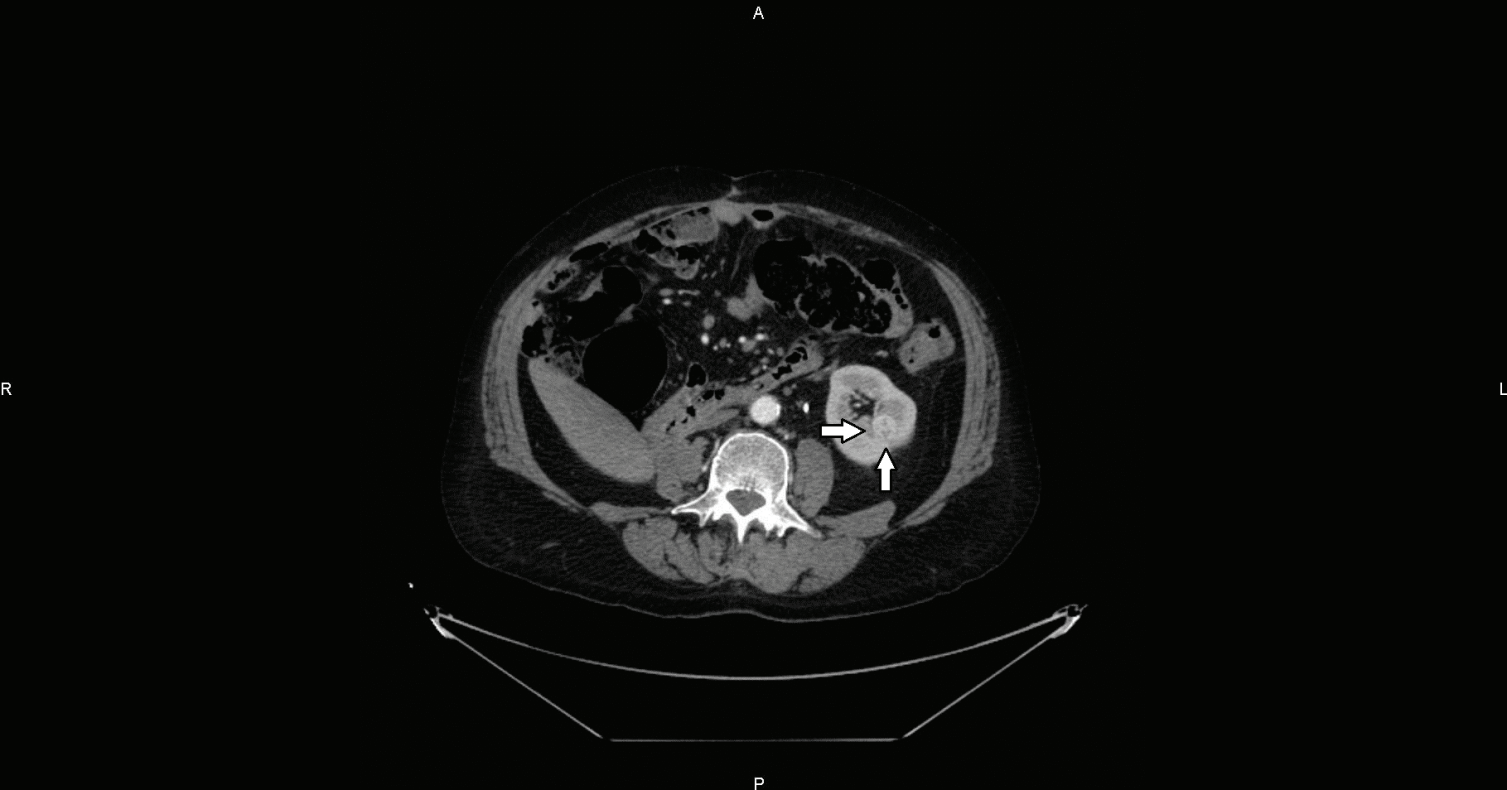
Axial CT image shows an intraparenchymal mid-renal hypervascular nodule referred to RCC (arrows).
